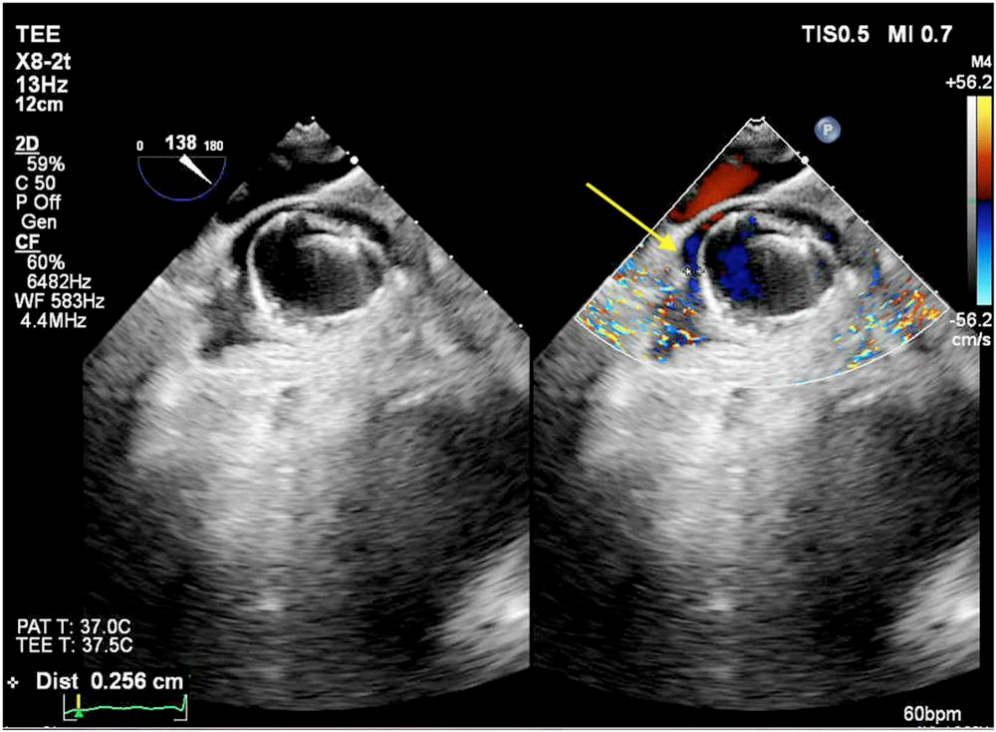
Transoesophageal echocardiogram showing incomplete occlusion of the left atrial appendage using a single 35 mm WATCHMAN device. Colour Doppler shows a >1 mm jet communicating between the anterior lobe and the left atrium.
